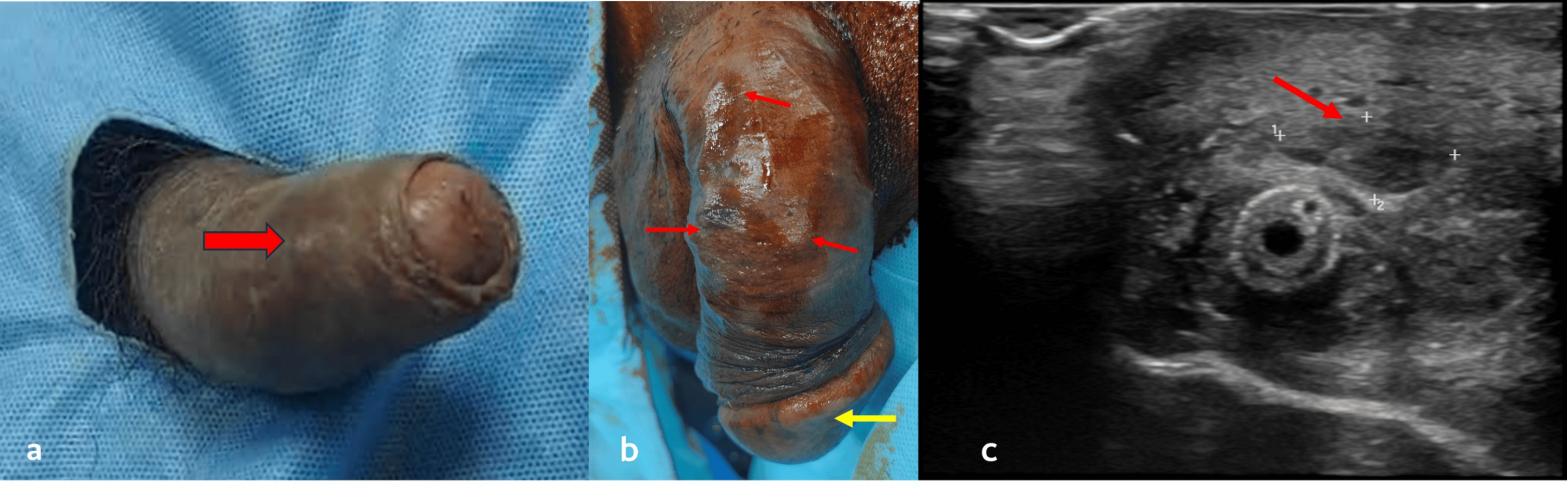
Clinical image (a and b) showing priapism and penile nodules, and ultrasonography (c) of the penis showing heteroechoic lesion Engorged penis with nodule over corpus cavernosum (arrow) (a), nodule over glans penis (yellow arrow), and multiple nodules over corpus cavernosum (red arrows) (b). Ultrasonography of the penis showing heterogeneous, predominantly hypoechoic lesion in the right corpus cavernosum (arrow) (c).
